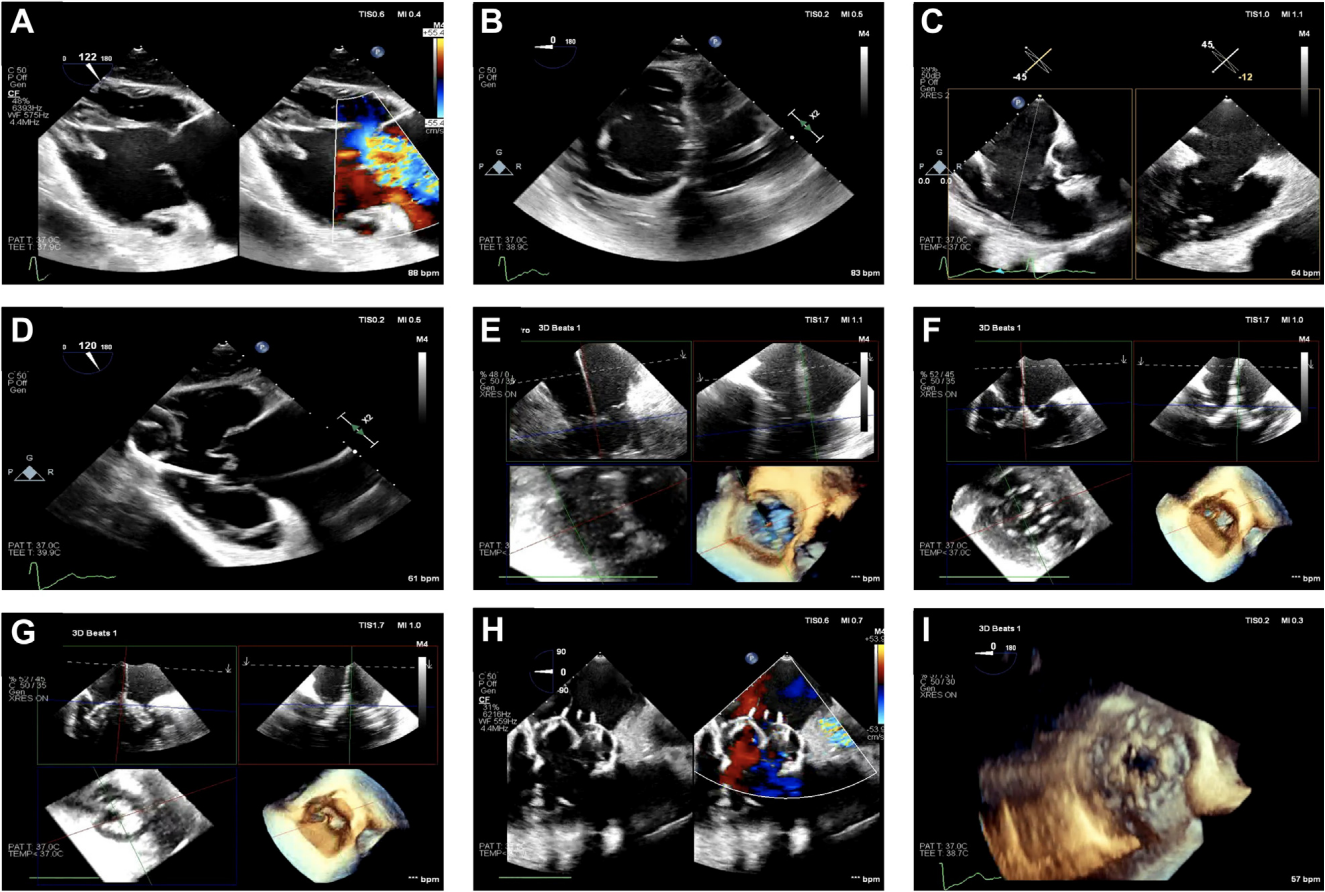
Visual Summary, Providing a Step-by-Step Imaging Guide to the Procedure (A) Baseline gastric long-axis view of the right ventricle (RV) with torrential tricuspid regurgitation (TR). (B) Short-axis view of the tricuspid valve with large coaptation gap. (C) Two-dimensional (2D) intracardiac echocardiography (ICE) demonstrating good visualization of the leaflets. (D) Two-dimensional (2D) transesophageal echocardiography and gastric images to guide wire placement in the RV apex and posteriorly. (E) 3D multiplanar reconstruction (MPR) on ICE being used to maneuver the delivery system into the RV. (F) Device anchors noted on 3D MPR by ICE; also noted are the 9 device anchors in the RV. (G) Ventricular expansion of the device. (H) After atrial expansion, results demonstrate no residual TR. (I) 3D image of the newly implanted EVOQUE device.
